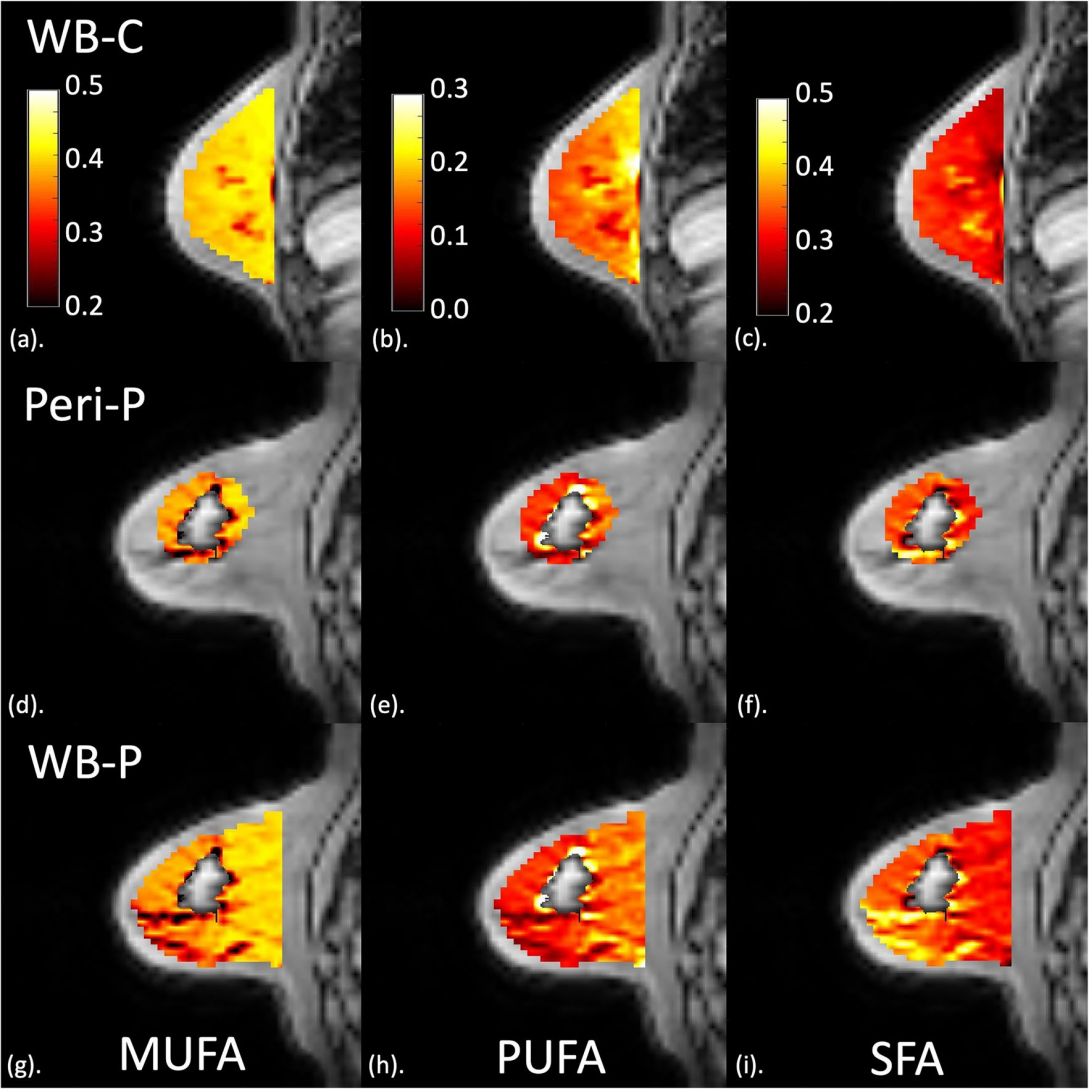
Peri-tumoural and whole breast lipid composition maps, obtained from MRI scans, in a typical participant (65-year-old woman with oestrogen receptor-positive breast cancer) and a control participant (overlaid on anatomical image). (a–c) MUFA, PUFA and SFA maps in the whole breast of a control participant (WB-C). (d–f) MUFA, PUFA and SFA maps in the peri-tumoural region of a participant (Peri-P). (g–i) MUFA, PUFA and SFA maps in the whole breast of a participant (WB-P). For the control participant, the mid-sagittal slice is shown. For the participant, the slice at the greatest dimension of the tumour (central grey area) is shown. *MUFA* monounsaturated fatty acids, *PUFA* polyunsaturated fatty acids, *SFA* saturated fatty acids.
